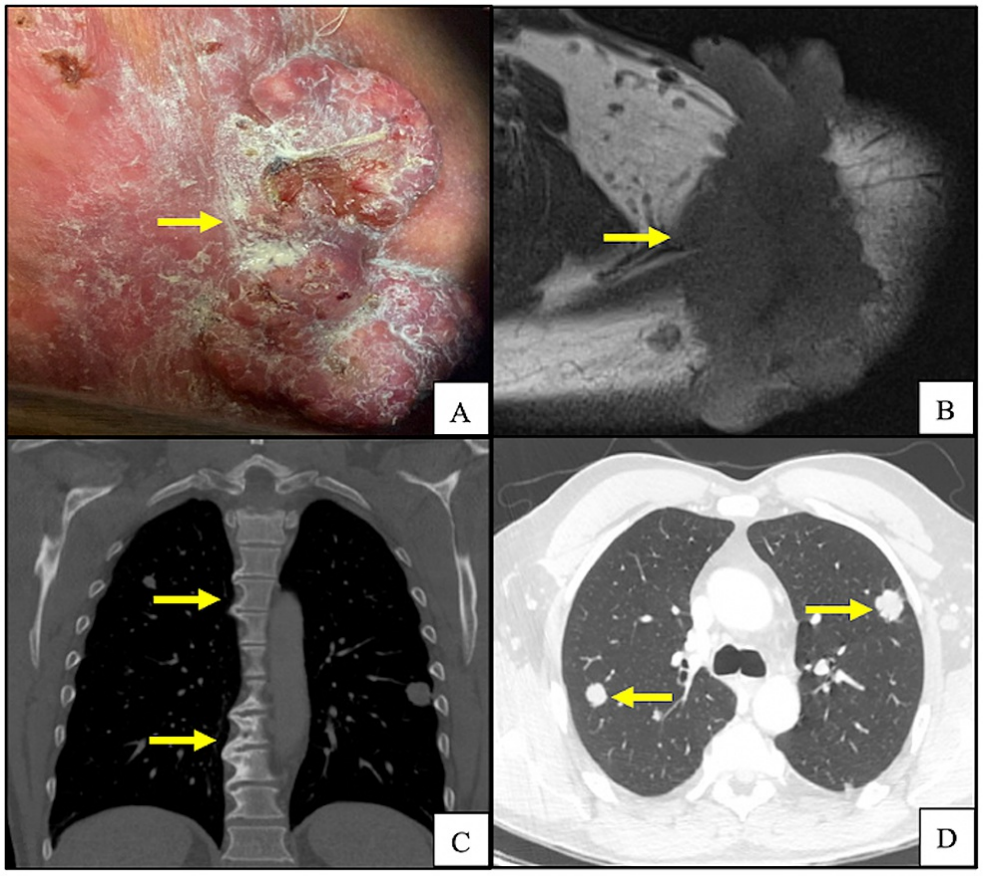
A) Gross pathology of BCC on the left shoulder at the time of diagnosis; B) axial MRI imaging of left shoulder at the time of diagnosis, image is indicative of invasive BCC; C) chest CT scan in bone window demonstrating sclerotic changes at T2/T3 and T6-T8 concerning for bony metastasis; D) chest CT scan in lung window demonstrating bilateral pulmonary nodules found to be metastatic BCC. Yellow arrows indicate abnormal pathology.
